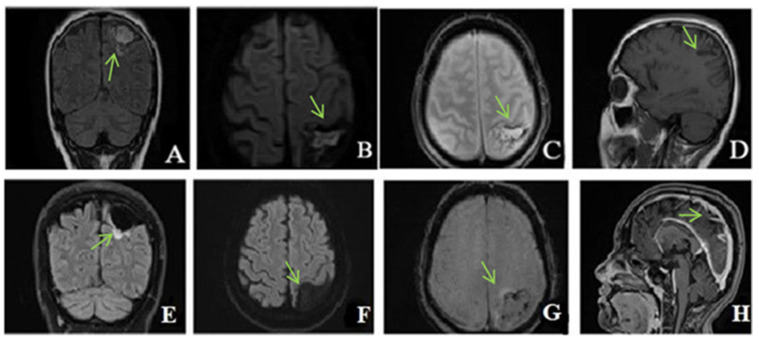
(A–D): Unenhanced brain MRI (at 3 months): (A)—Fluid attenuated inversion recovery (FLAIR) coronal, (B)—Diffusion-weighted imaging (DWI) axial, (C)—T2* hemo axial, (D)—T1 sagittal: Chronic ischemic left parietal lesion with hemorrhagic transformation partially resolved. E-H: Unenhanced and enhanced brain MRI (at six years): (E)—FLAIR coronal, (F)—DWI axial, (G)—T2* hemo axial, (H)—T1 sagittal after i.v. contrast administration: Chronic ischemic left parietal lesion with slightly peripheral gliosis, without restricted diffusion and minimal hemosiderin peripheral deposits, without filling defect of the veins and venous sinuses.
